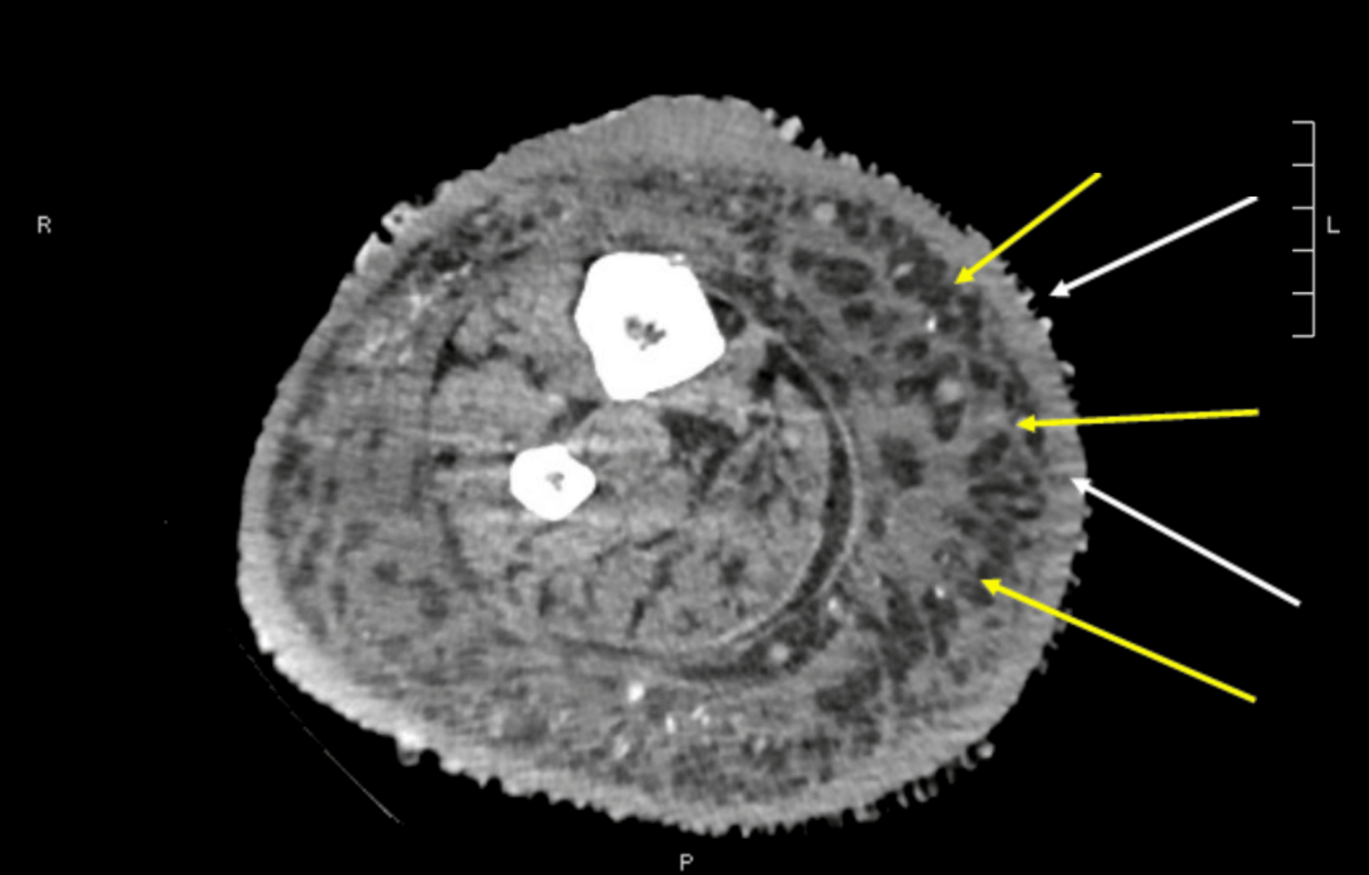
CT scan of the right lower extremity demonstrating hyperkeratosis (white arrows) and subcutaneous bullae (yellow arrows), characteristic features of ENV. ENV: Elephantiasis nostra verrucosa.
